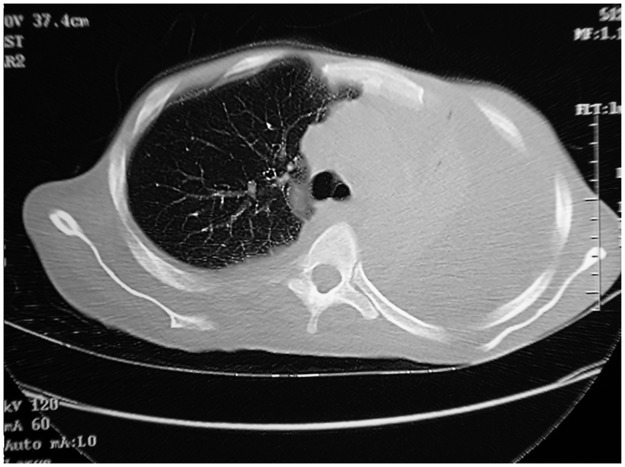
Chest CT scan showing a massive left pleural effusion with collapsed left lung.
